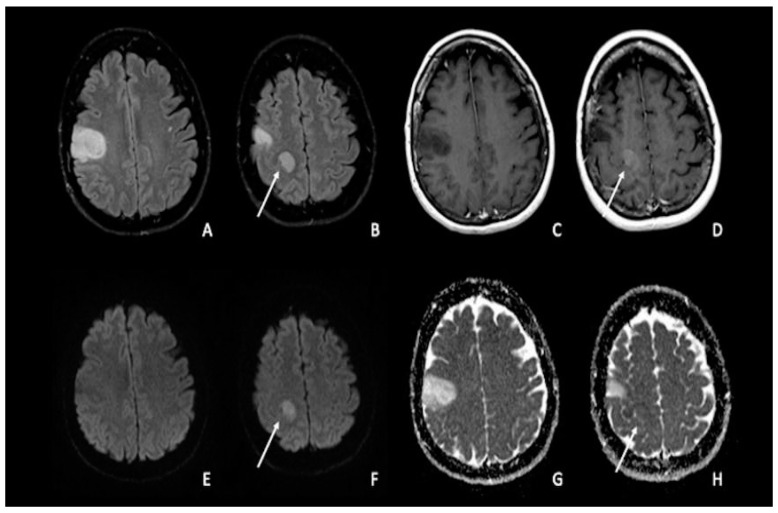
Double site of glioma infiltration in the right Rolandic frontal region: (A,B) Both lesions present similar hyperintensity on FLAIR images. The most cranial lesion (arrow) showed slight post-contrastographic enhancement (D) and restricted water diffusion on DWI (F), characterized by slightly low values on ADC map (H); this condition could be linked to an anaplastic aspect of the tumor, with restricted diffusion explained by an increase in tumor cellularity. The most caudal lesion did not show contrast enhancement, with a marked hypointense signal on post-contrastographic T1-weighted image (C); the same lesion presented no restricted diffusion with increased ADC values (E–G). FLAIR = fluid-attenuated inversion recovery; DWI = diffusion-weighted imaging; ADC: apparent diffusion coefficient.
